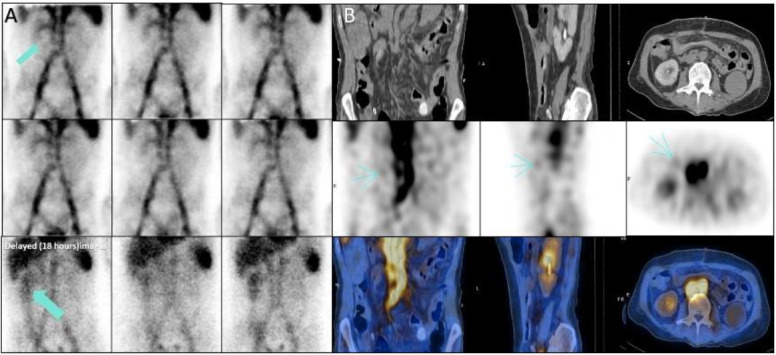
Planar and SPECT/CT images localized the source of bleeding to the anastomotic area (green arrows A, B)
